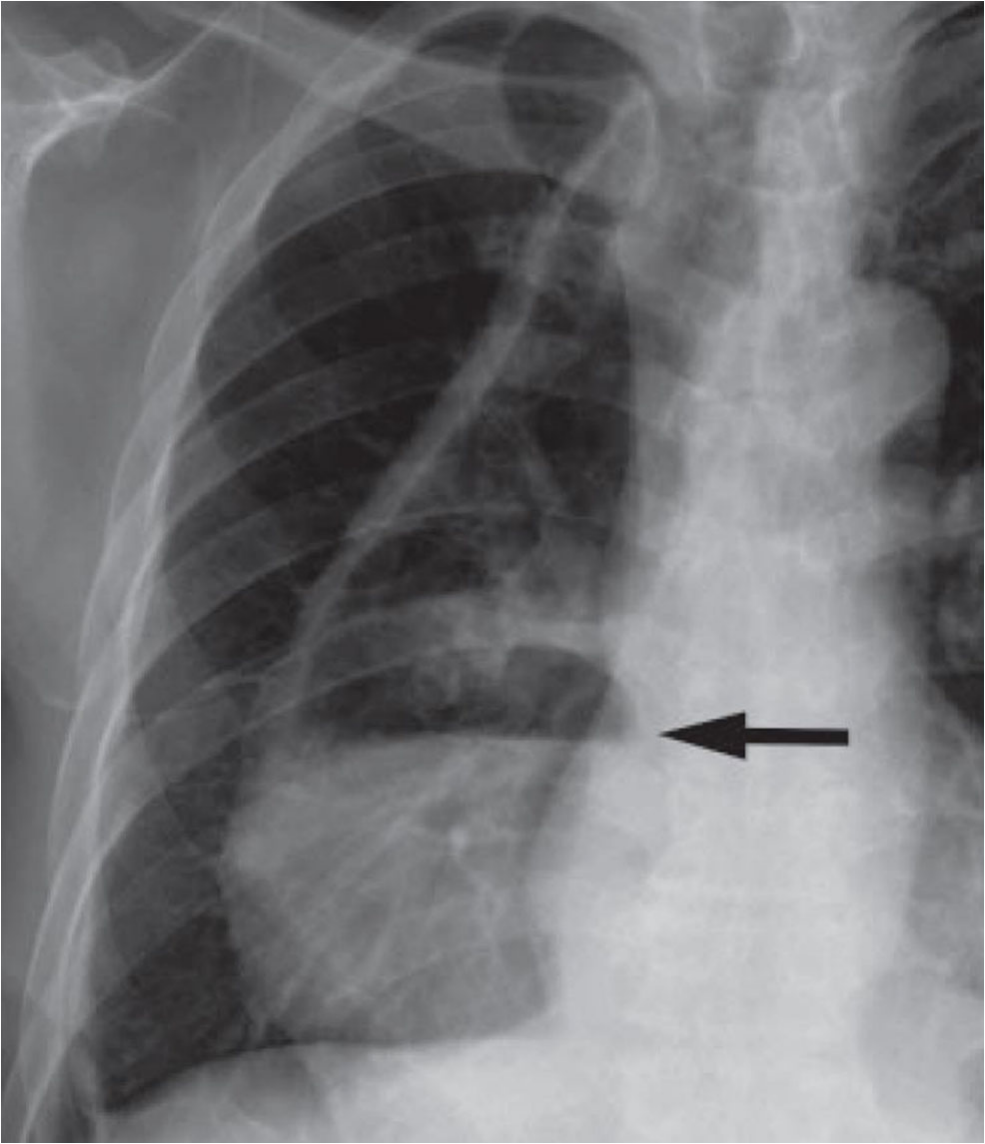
Plain chest X-ray showing a dilated gastric conduit with air-fluid level in a patient with DGE after esophagectomy and gastric conduit reconstruction
